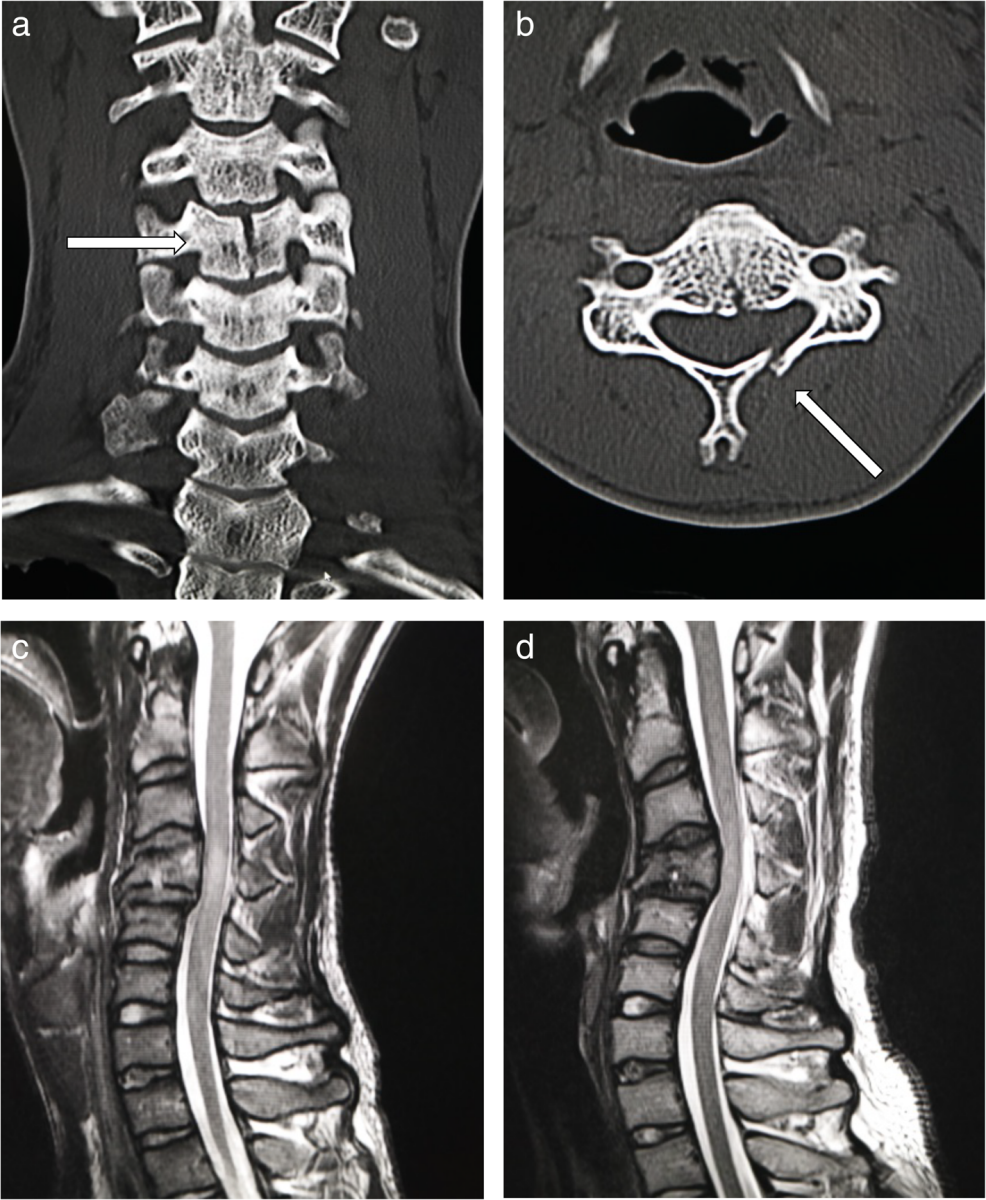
A representative case with progression of local kyphosis. a, b Computed tomography (CT) images at the time of injury showed the vertebral vertical fracture and left laminar fracture at the C4 level. c Cervical spinal cord injury was confirmed by T2-weighted magnetic resonance imaging (MRI) at the time of injury. d MRI at the time of final follow-up (1110 days after injury) showed the progression of local cervical kyphosis from 17 to 36°, measured by the endplate method. His neurological finding remained at AIS grade D and improved to modified Frankel grade D3
